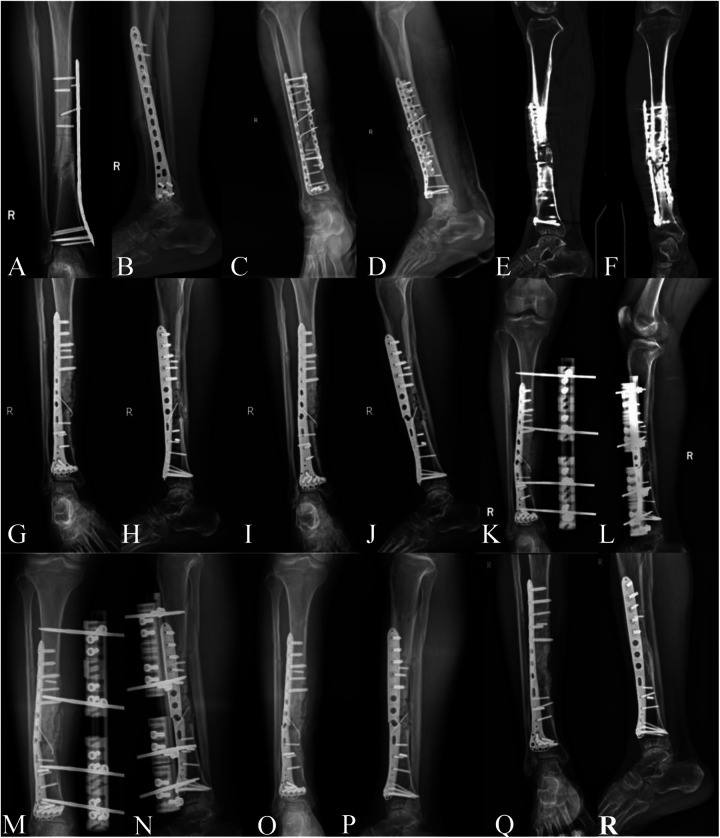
(A,B) Internal fixation failure and nonunion observed 9 months post-first surgery. (C,D) x-ray images on the day of the second surgery. (E,F) Preoperative CT showing bilateral plate failure and nonunion before the third surgery. (G,H) x-ray images on the day of the third surgery. (I,J) Preoperative x-ray images of the fourth surgery showing plate failure with external callus wrapping the fracture site, good alignment of the fracture. (K,L) x-ray images on the day of the fourth surgery. (M,N) Preoperative x-ray images for the fifth surgery. (O,P) Postoperative images after the fifth surgery, showing removal of external fixator, plate failure, and atrophic nonunion. (Q,R) 11.3 months post-sixth surgery, outpatient imaging shows indistinct fracture line, cortical bone bridge formation, and good tibiofibular alignment.
